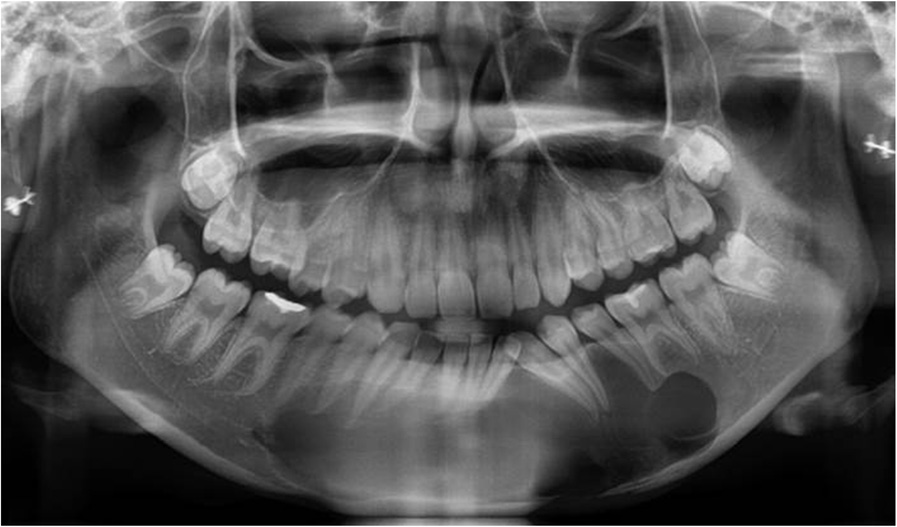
Radiographic findings at the initial visit. A well-defined radiolucent lesion is observed in the mandible. The roots of the tooth in the lesion were not resorbed, but the displacement of tooth was evident
